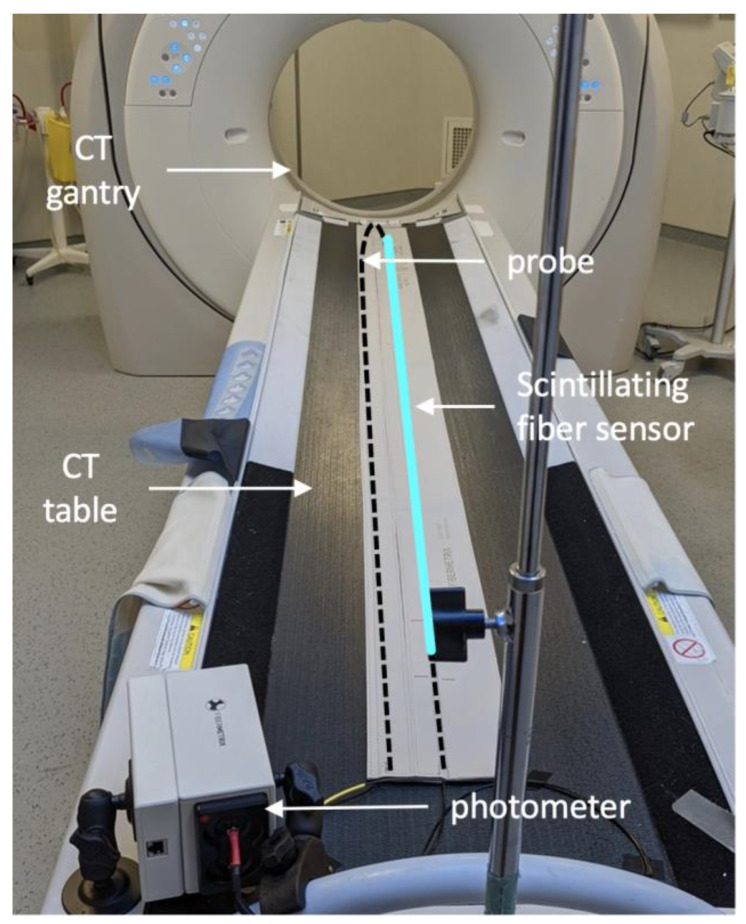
IVIscan system installed on a CT scan. The probe is placed on the CT table forming a U-shape at the head side of the table (black dotted line) and is connected to the photometer fixed at the feet side of the table. The scintillating fiber sensor (blue line) is placed in line to measure air kerma all along the exposure area on the CT table.
